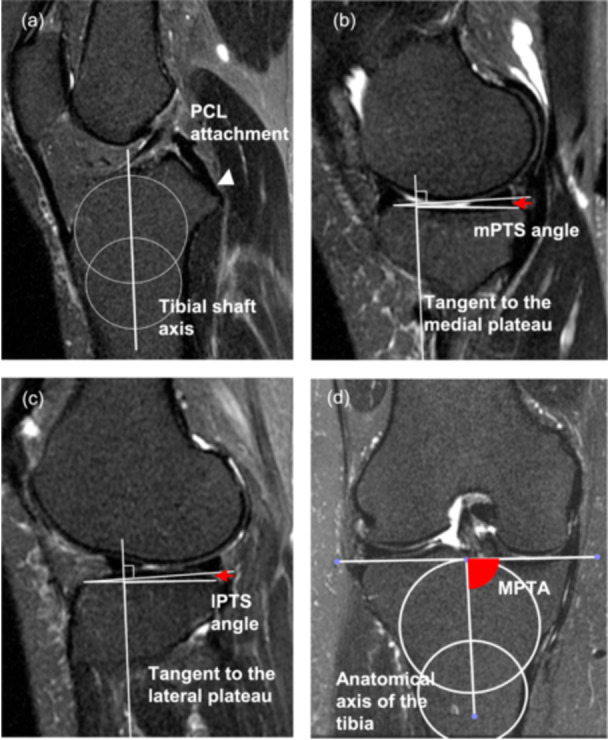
Measurements of proximal tibial morphological parameters performed using MRI. The central sagittal plane is shown on the slice corresponding to the attachment site of the posterior cruciate ligament and the intercondylar eminence. On this slice, two circles are fitted to the anterior and posterior cortices of the tibia, and the line connecting the centres of these circles is defined as the tibial axis (a). The medial and lateral posterior tibial slopes (mPTS and lPTS) are calculated as the angles between a line perpendicular to the tibial axis and the line connecting the most proximal anterior and posterior subchondral bone points at the centres of the medial and lateral tibiofemoral compartments, respectively (b and c). The medial proximal tibial angle (MPTA) is defined as the angle formed between the anatomical axis of the tibia and the proximal tibial joint line on the coronal MRI view (d). MRI, magnetic resonance imaging.
